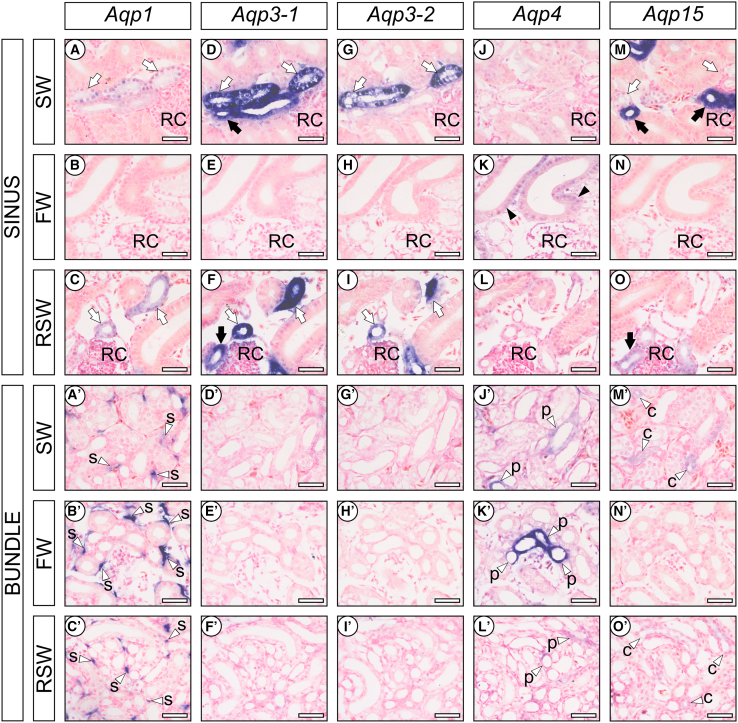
In situ* hybridization analysis on *Aqp*s in the red stingray nephron The respective mRNA signals in sinus zone (A–O) and bundle zone (A′ –O′) of *Aqp1* (A–C and A′–C′), *3-1* (D–F and D′–F′), *3-2* (G–I and G′–I′), *4* (J–L and J′–L′), and *15* (M–O and M′–O′) are shown. Filled arrowheads indicate the PII (K). Open and filled arrows indicate the anterior and posterior LDT, respectively (A–I and M–O). RC, renal corpuscle. Open arrowheads indicate the peritubular sheath (labeled with “s”), PI (labeled with “p”), and CT (labeled with “c”) in the bundle zone (A′-B′ and J′-O′). Scale bars, 50 μm. Note that (1) in the sinus zone of FW-acclimated stingray, the mRNA signals of *Aqp1*, *3-1*, *3-2*, and *15* were almost undetectable, while (2) in the bundle zone, intense mRNA signals of *Aqp1* and *4* were observed in the peritubular sheath and PI of FW-acclimated stingrays, respectively, compared with SW control and RSW-acclimated individuals.
